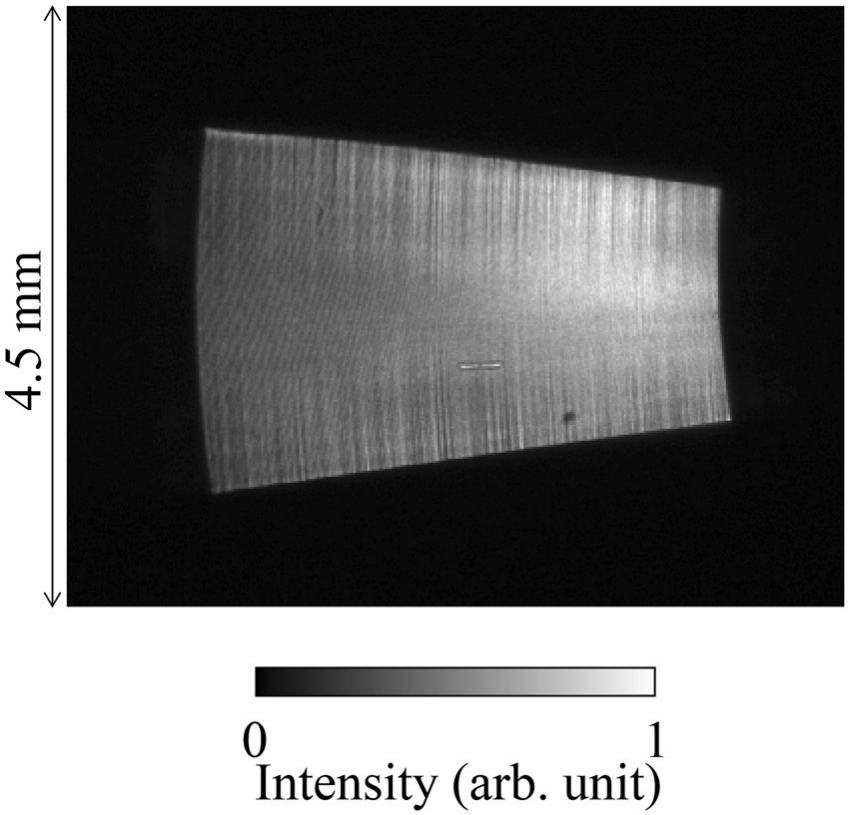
Intensity distribution reflected from ellipsoidal focusing mirror. The image is observed at X-ray energy of 7 keV using a two-dimensional X-ray detector located 0.9 m downstream from the focus. The vertical and horizontal directions in the figure correspond to the sagittal and meridional focusing directions.
